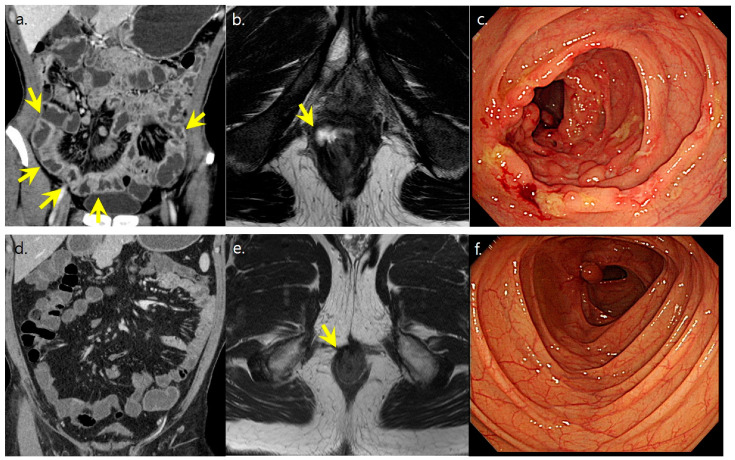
A 36-year-old male with Crohn’s disease showing well-controlled disease course. (a,b) Initial CT and MR showed active inflammation in the entire ileum and colon with perianal abscess. (arrows) CT scores was graded as severe activity. (c) Initial SES-CD score was graded as severe with multiple ulcers, exudates, erythema, and cobble stone appearance in the colons. (d,e) Two-year follow-up CT showed resolved active inflammation in both bowel loops and perianal region (arrows in (e)), and CT scores was graded as no activity. (f) Patient was in clinical and endoscopic remission at the last follow up.
